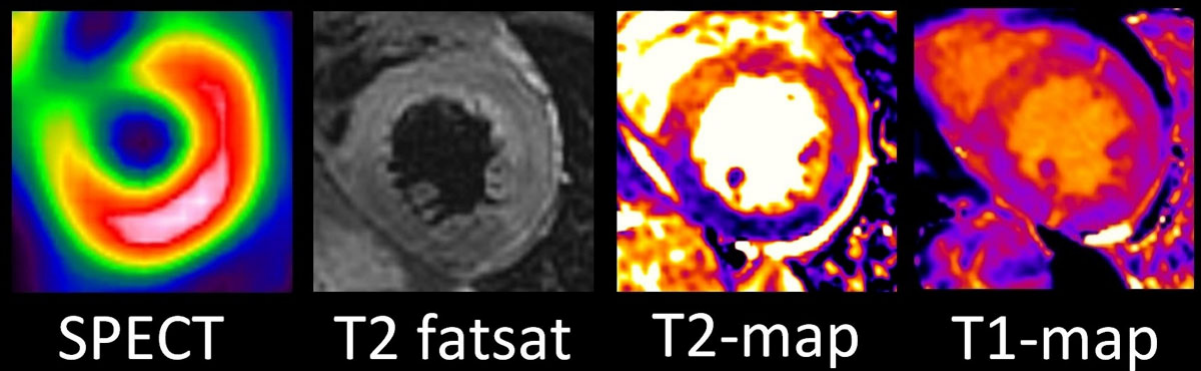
Area at risk in a corresponding short axis slices in SPECT, T2 fatsat, T2-map and T1-map images (from left to right).
